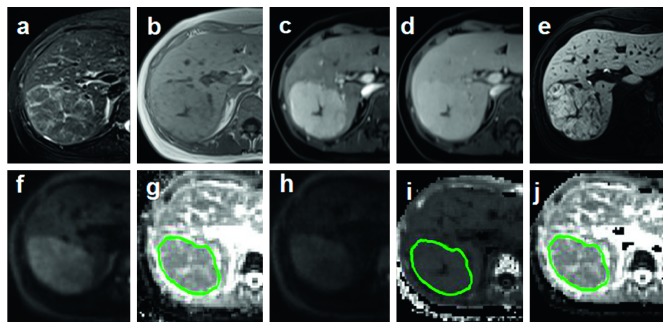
ROI was drawn on the level of maximum transactional diameter of FNH (green circle). (a, b) Axial fat suppress T2WI and T1WI. (c–e) Dynamic enhancement imaging included artery phase, portal phase, and hepatobiliary phase (HBP). (f, g) Axial liver DWI (*b* = 800 s/mm^2^) and ADC maps. (h–j) Axial liver DKI (*b* = 2000 s/mm^2^) and DKI-derived parameters *K* and *D* maps.
